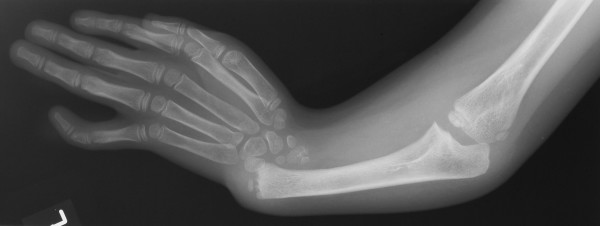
AP radiograph of the upper limb showing absent radius with radially deviated hand.
